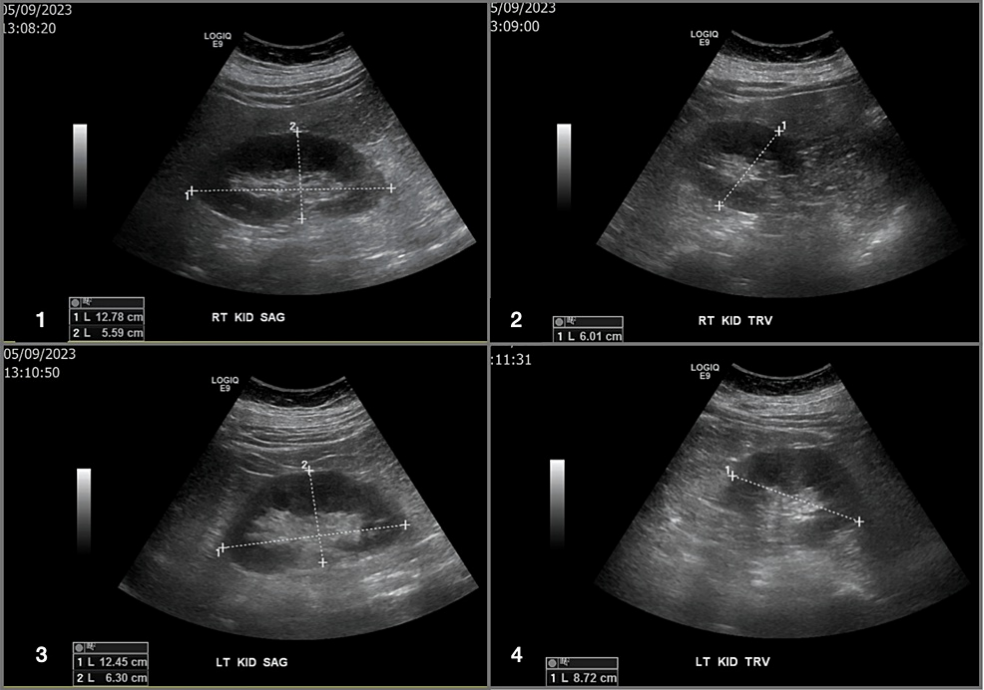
Right and Left Renal Ultrasound. 1- Right Kidney Sagittal View 2- Right Kidney Transverse View 3- Left Kidney Sagital View 4- Left Kidney Transverse View
